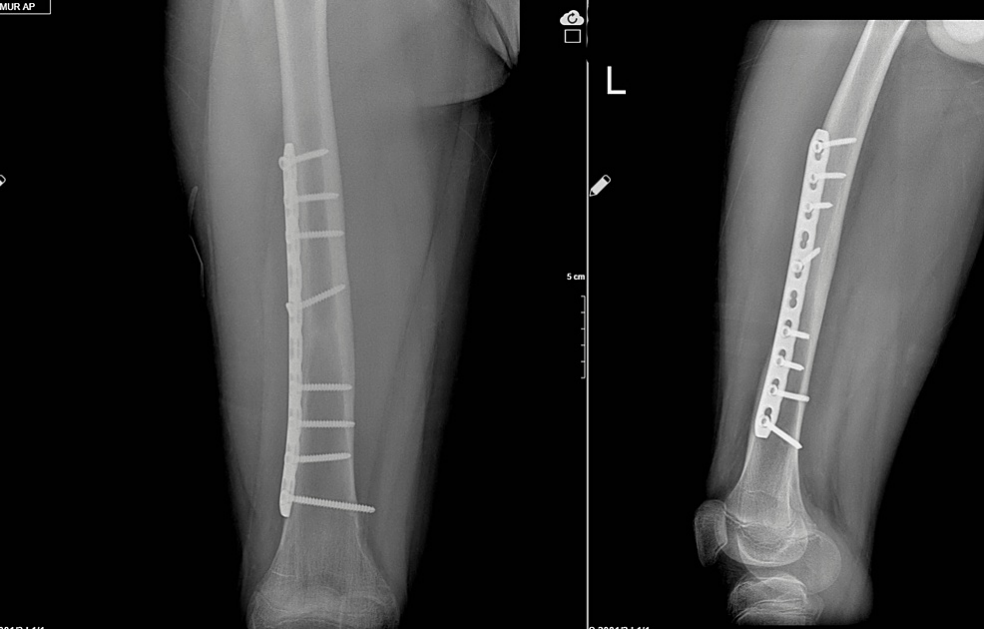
Treated with plate-screw osteosynthesis. Six months follow-up radiographs show the union of the fracture site.
